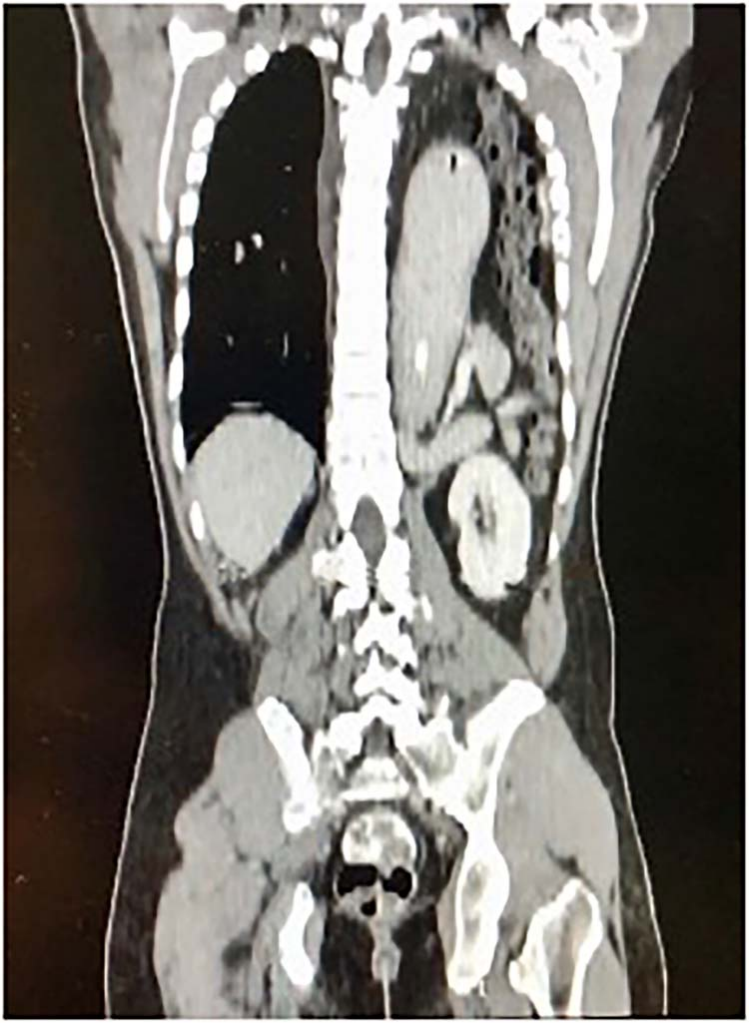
Longitudinal thoraco-abdominal CT scan shows protrusion of stomach, small and large bowel through a left posterolateral hemi-diaphragmatic defect.
